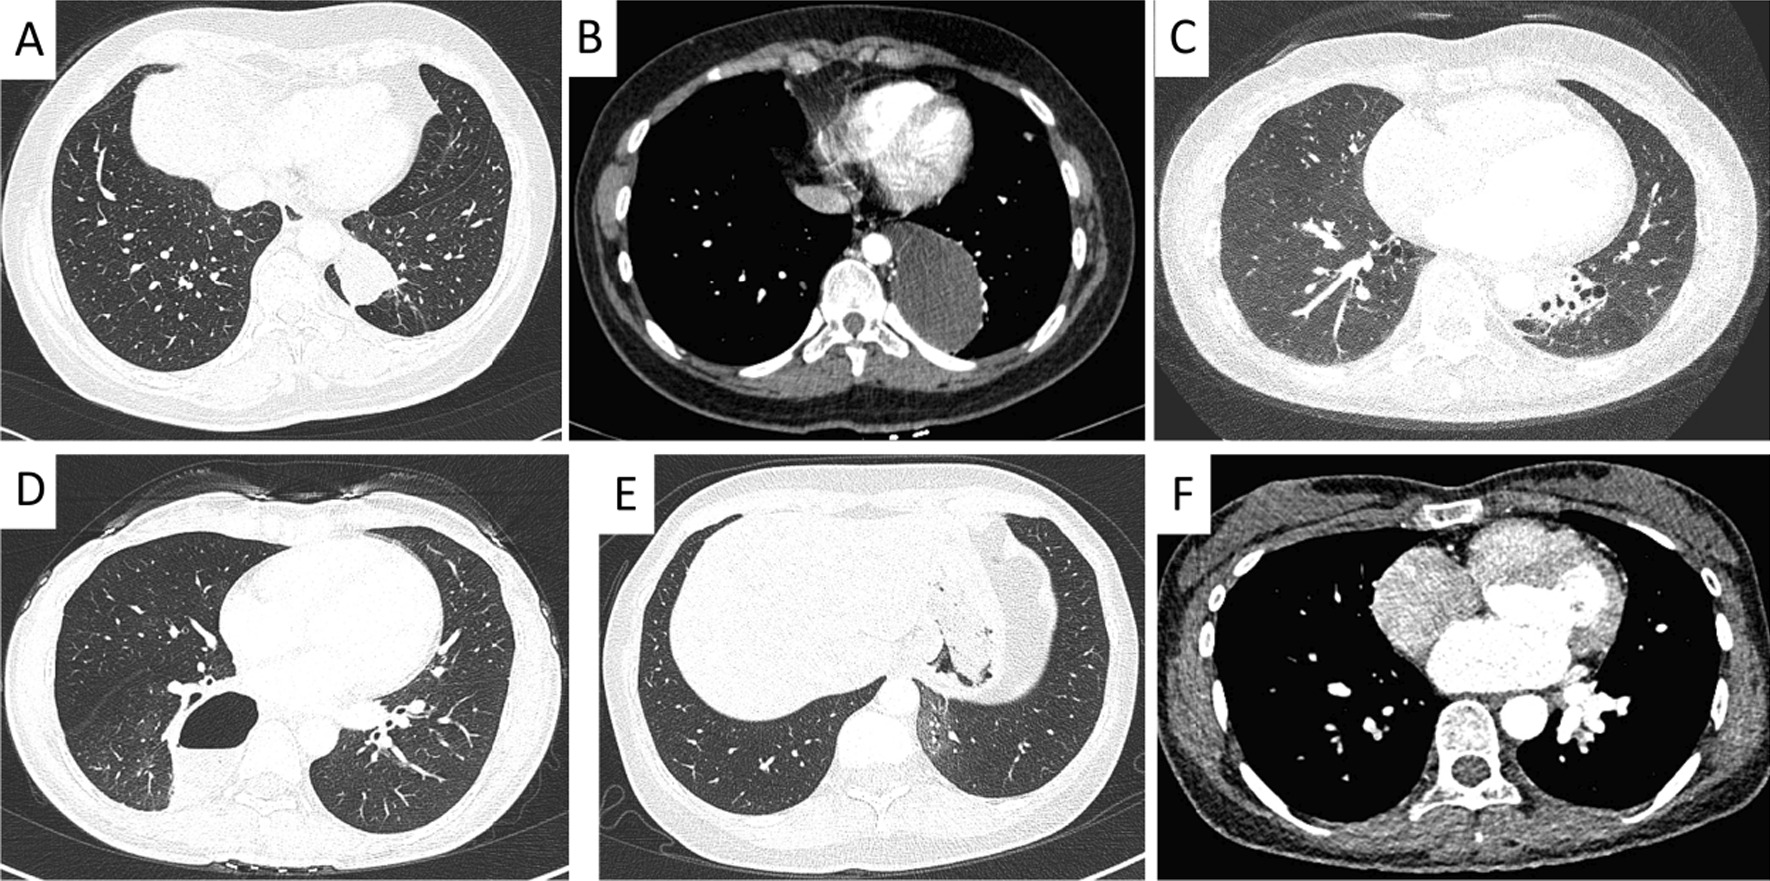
Representative CT appearances of pulmonary sequestration. A masse lesions; B cystic lesions; C bronchiectasis; D cavitary lesions; E pneumonic lesions; F intrapulmonary cord-like shadow
